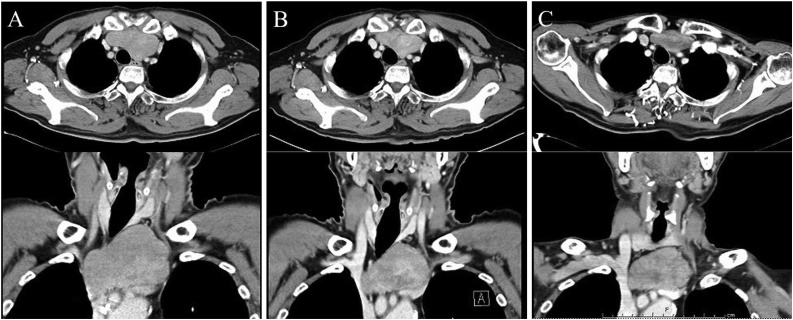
Change in tumor diameter on CT follow-up. The tumor size regressed from 7.3 × 4.3 × 5.2 cm according to the CT performed eight years ago (A) to 6.5 × 3.0 × 5.0 cm by CT carried out four years ago (B) and 5.7 × 2.7 × 4.0 cm in the latest CT taken before surgery (C).
